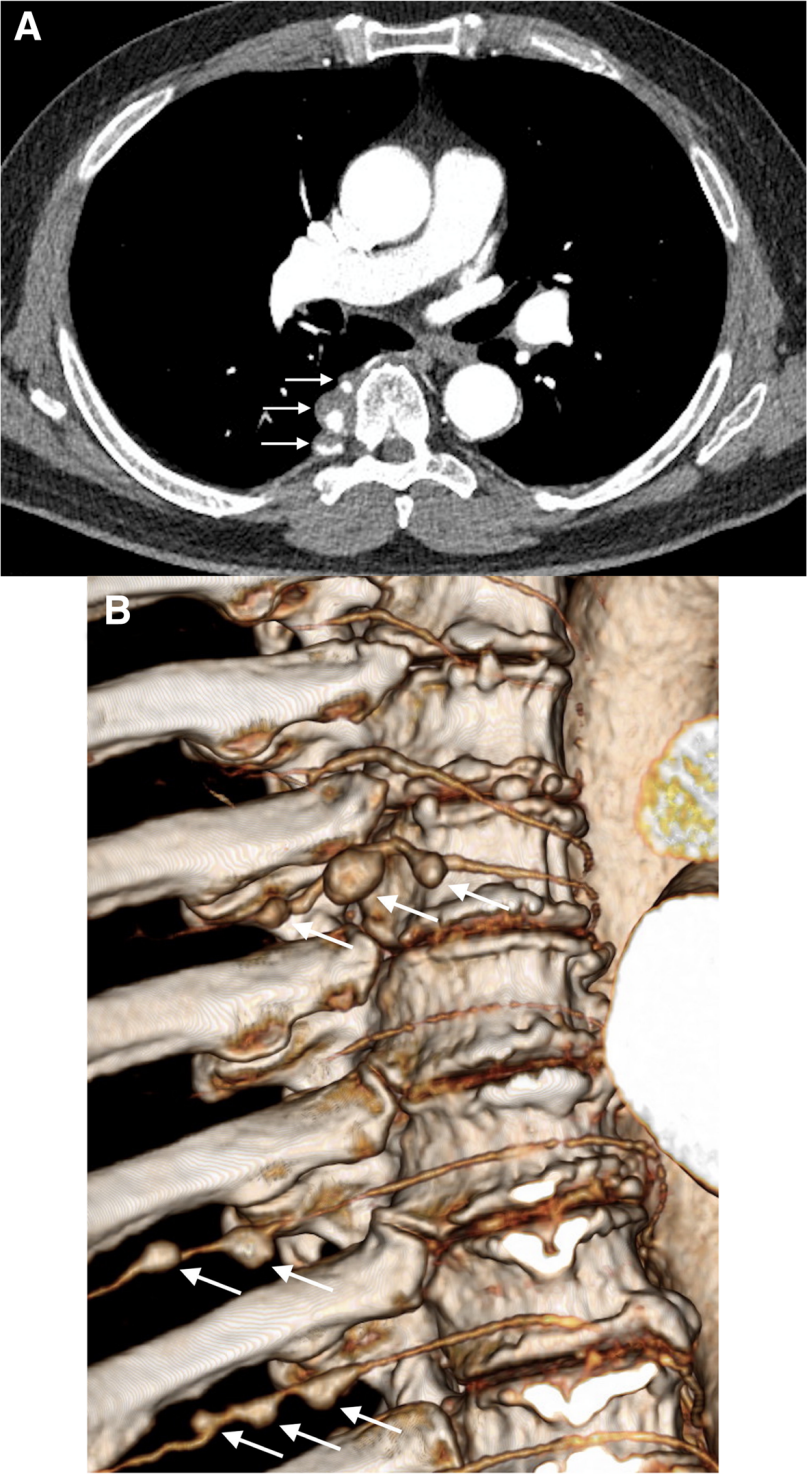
CT angiography. a Axial CT image shows three dense nodules and eccentric lower attenuation material (arrows) consistent with thrombus containing aneurysms of the proximal right 7th intercostal artery, the largest measuring 2.1 cm × 1.8 cm. b Three-dimensional reconstruction shows multifocal aneurysmal dilatations at multiple levels (arrows)
